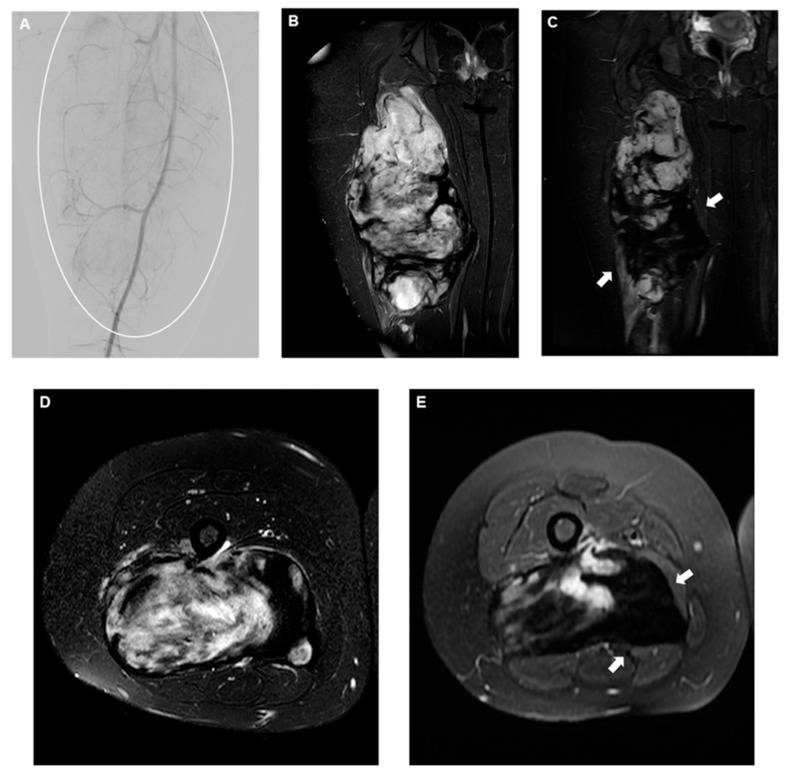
(A) DSA of the superficial femoral artery showing a slightly vascularized large mass encompassing the majority of the leg (circle), and (B) coronal and (D) axial T2-fat saturated pre-procedural MRI demonstrating the classic appearance of a very active desmoid fibromatosis, mostly characterized by high-intensity T2 signal areas. Four-month follow-up coronal (C) and axial (E) T2-fat saturated MRI showing a significant decrease in T2 signal intensity (arrows), corresponding to inactive disease following embolization. Embolic material used was 300–500 microns microspheres.
